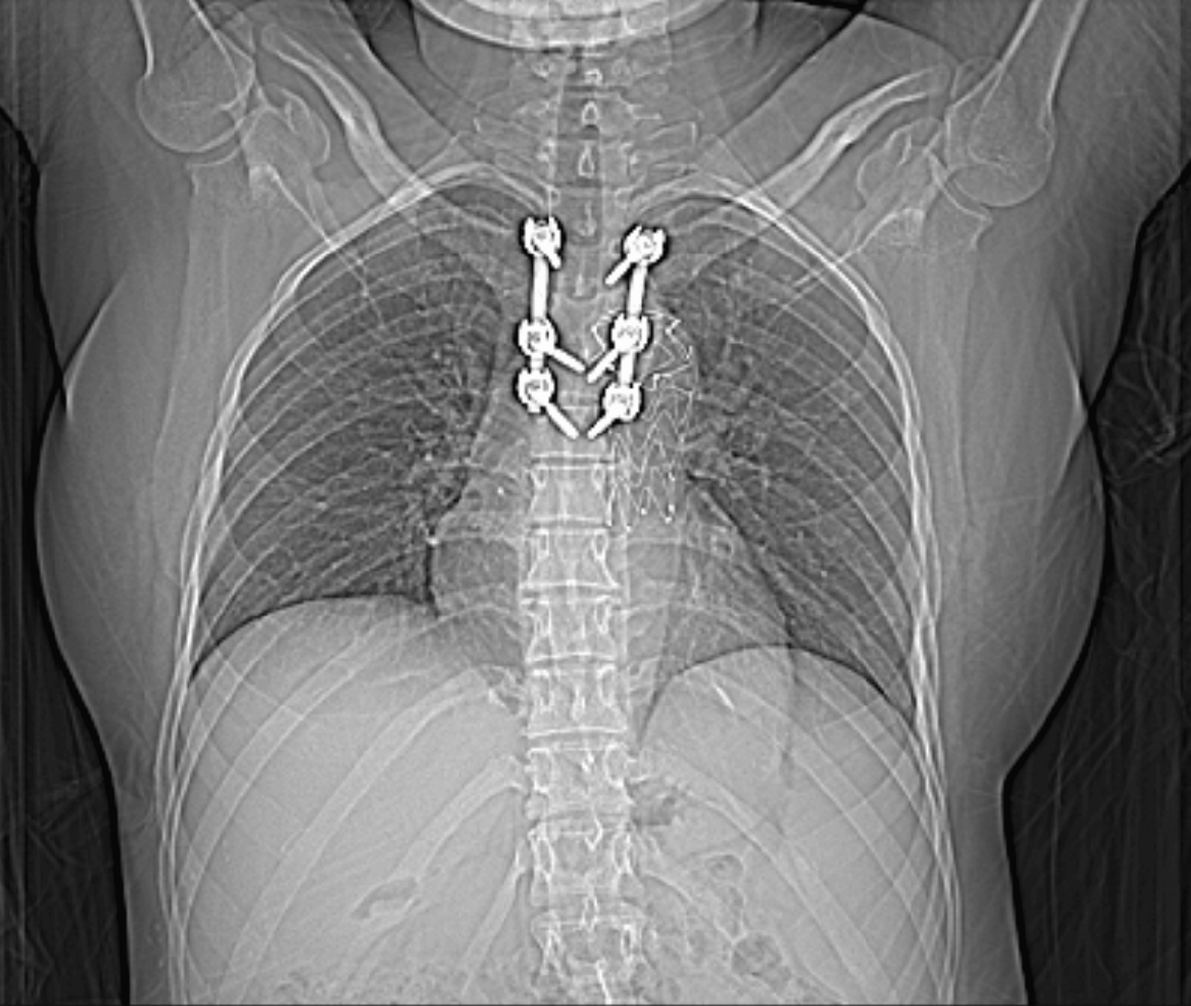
CT scan Aortic stent-graft in place with posterior instrumentation of the spine
